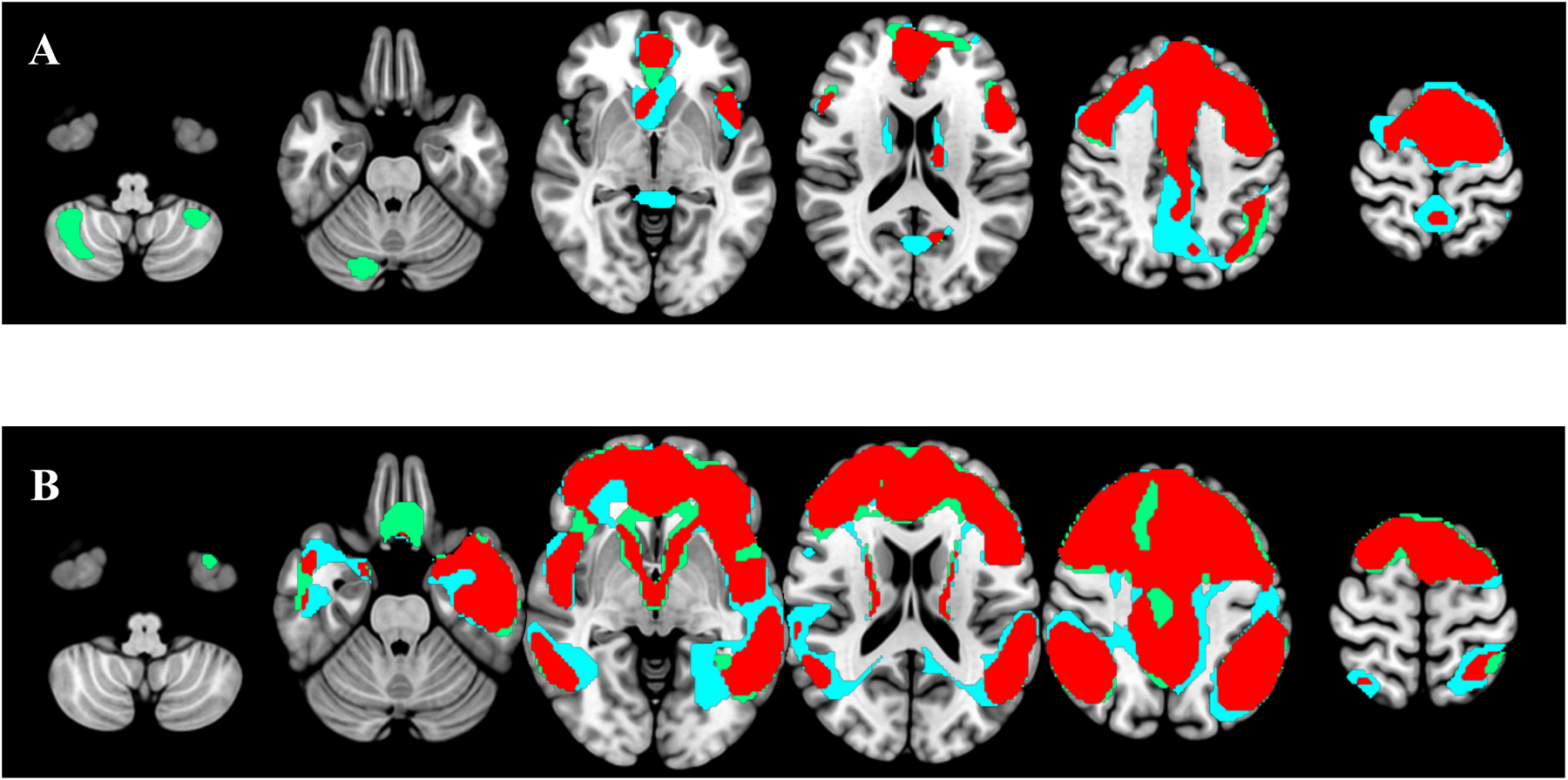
Hypoperfusion and hypometabolic patterns and their overlap at the single-subject level. In blue, eFBB SPM binarized t-map. In green, FDG SPM binarized t-map, and in red, the overlap of both images. The standard Montreal Neurological Institute template was used to overlay the hypometabolism and hypoperfusion maps, along with their overlap. A) Patient with suspected AD and negative lFBB. Hypoperfusion and hypometabolic patterns are not indicative of neurodegeneration in AD. Dice index 0.76. B). Patient with suspected Alzheimer's disease and positive lFBB. Hypoperfusion and hypometabolic patterns are compatible with AD. Dice index 0.79 (colors are visible in the online version).
